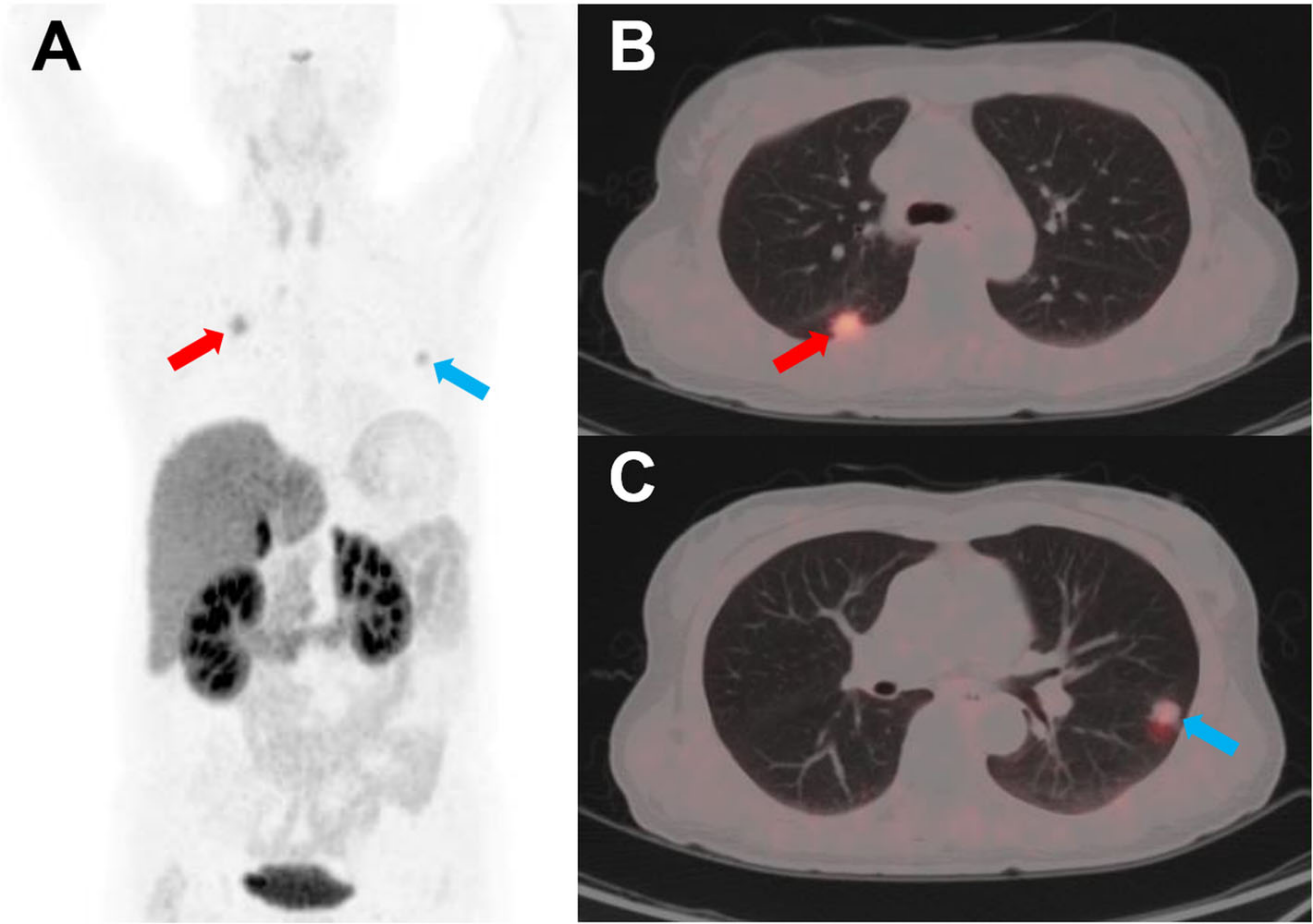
Representative case of a 55-year-old male with a previous primary pancreatic NET grade 2. The patient underwent surgery for the primary pancreatic NET 8 years prior, and multiple lung nodules were found in a follow-up chest CT image. MIP (a) and trans-axial (b, c) [^68^Ga]Ga-DOTA-TOC PET/CT images demonstrate small metastases with moderate uptake (red and blue arrows) in both lungs. His low TLR (0.98) and low SUVmax (5.85) predicted a poor prognosis; however, his good ECOG performance status (0) and low WTV (0.0) predicted a good prognosis. After lanreotide therapy, disease progression (metastatic nodule) was found in the right lower pulmonary lobe (red arrow; long-diameter increased from 1.2 to 1.7 cm) after 3 months of follow-up
